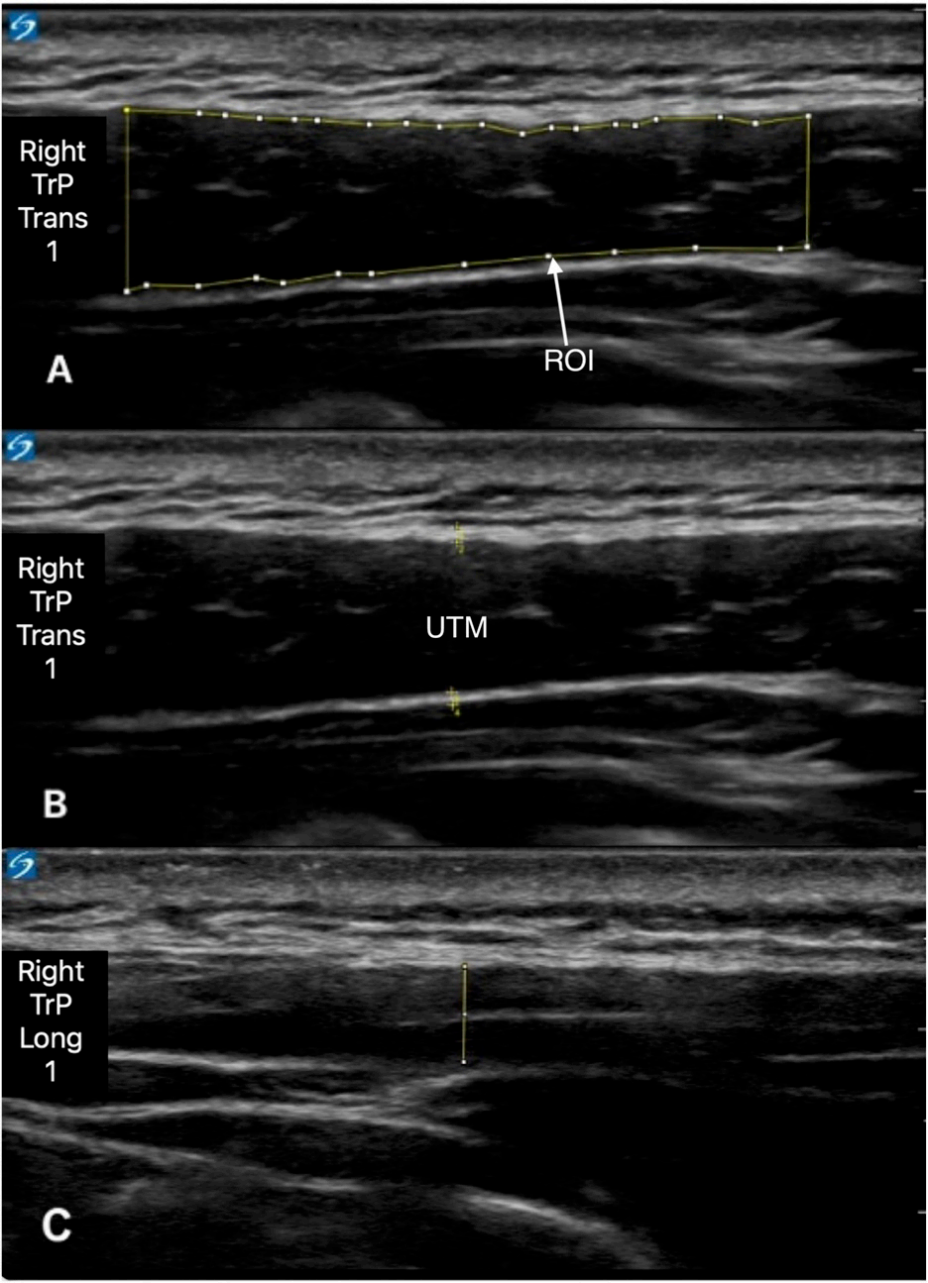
Ultrasound Image of the UTM [Upper Trapezius Muscle] in Transversal (A + B) and Longitudinal (C) orientation A: Selection of the ROI [Region of Interest] for Gray Scale Analysis; ROI Were Set by Selecting the UTM Muscular Tissue in the Approximated Central 75% of the Picture’s width. B: Measurement of Upper and Lower Fascial thickness. C: Measurement of Muscle Belly Thickness (Image Editing by FIJI Version 1.54f). Abbreviations: TrP (Myofascial Trigger Point), Trans (Transversal), Long (Longitudinal)
